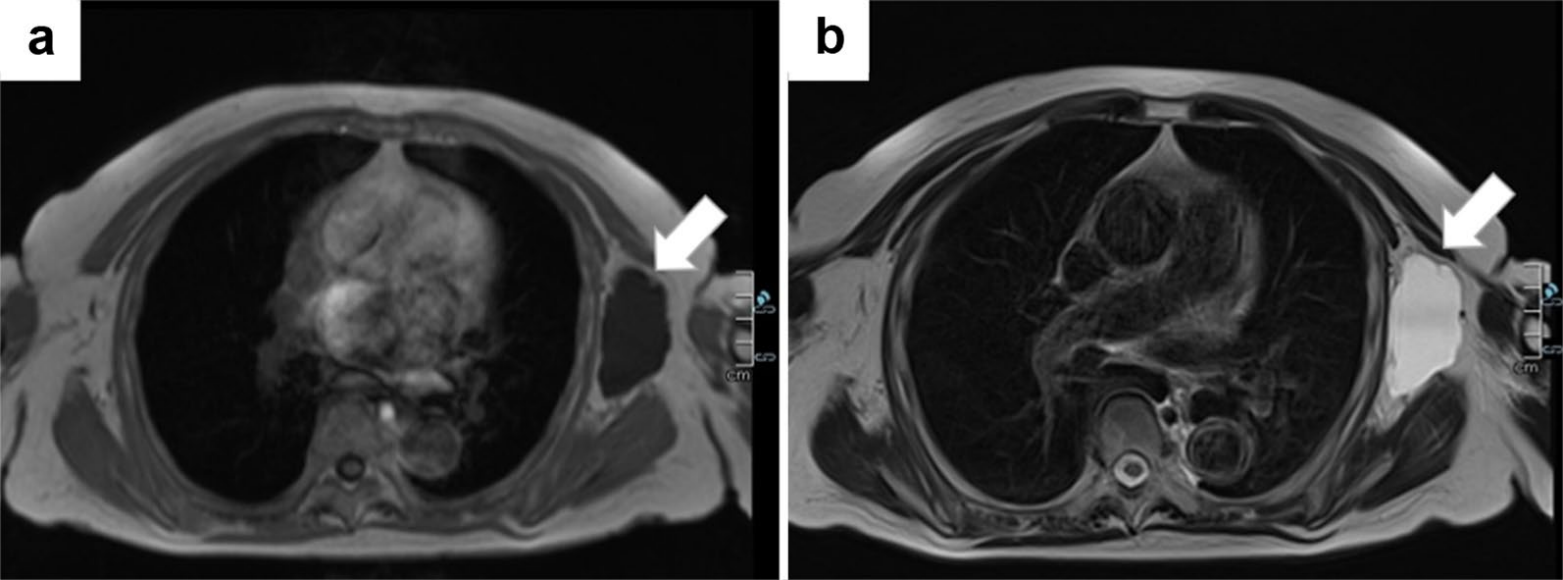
Magnetic resonance imaging (MRI) findings. Compared with the surrounding muscle, the left axillary mass showed a low signal on T1-weighted imaging (a) and high signal on T2-weighted imaging (b)
